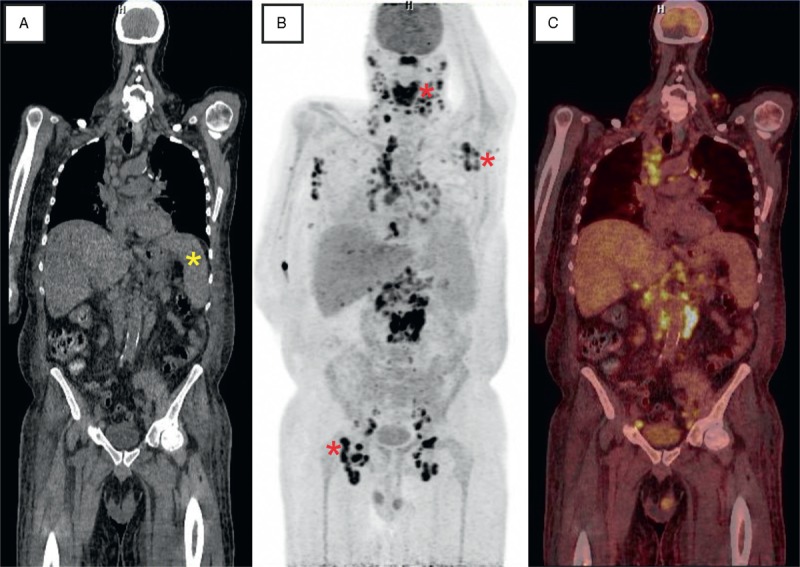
Slices of fluorodeoxyglucose PET combined with computed tomography (A: CT; B: PET; C: fusion of PET/CT). Besides a splenomegaly (yellow asterisk) a very intensive uptake was measured in nearly all lymph node stations. In particular, pathologic [^18^F]2-fluoro-2-deoxy-D-glucose accumulation was detected in cervical, axillary, mediastinal, paraaortic, and inguinal lymph nodes (red asterisks) as well as in the tongue, thyroid, and lung (MIP–PET). CT = computed tomography, MIP = maximum intensity projection, PET = positron emission tomography.
